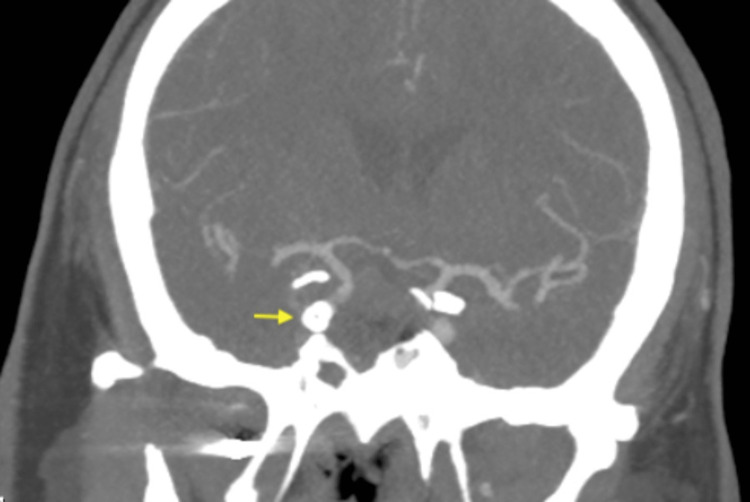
CTA coronal MIP image showing the right internal carotid artery flow diverter (yellow arrow) with filling of the distal internal carotid artery.
